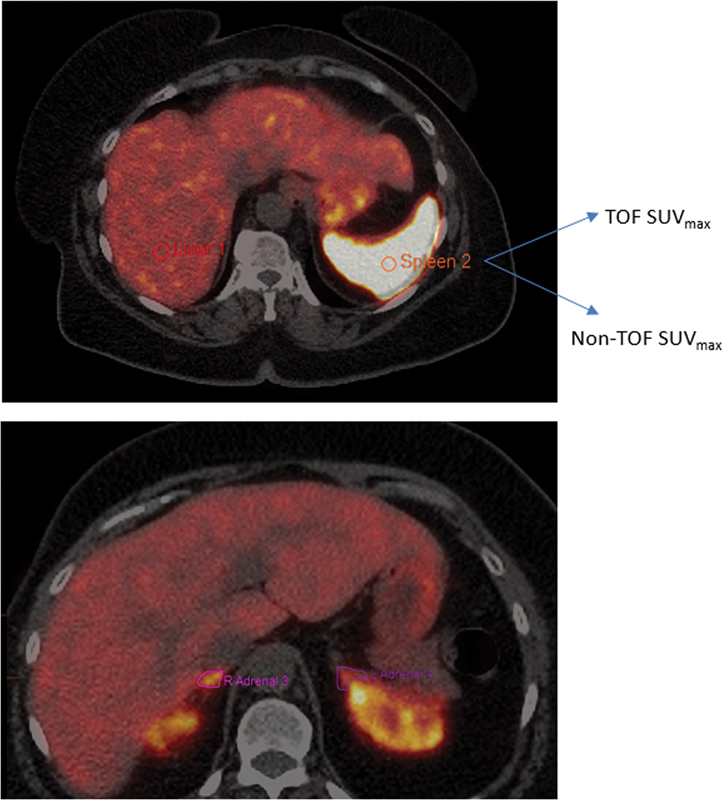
A one centimeter square (cm2) region of interest (ROI) was drawn on an anatomically normal appearing area of 24 preferentially chosen structures to measure maximum standardized uptake value (SUV
